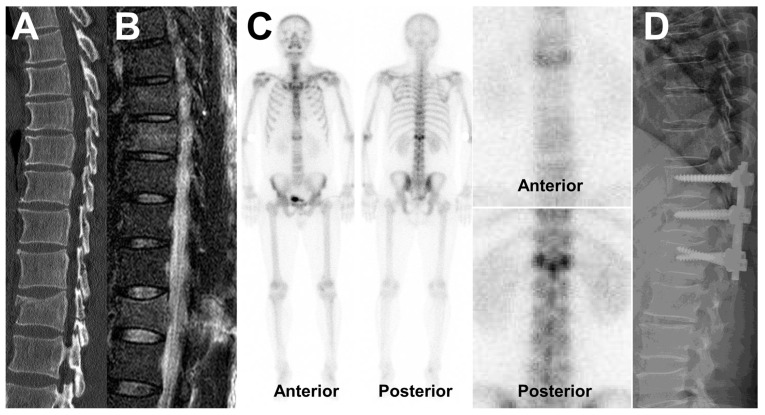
Representative images of a burst fracture of T12 in a 44-year-old patient. (A) Computed tomography and (B) magnetic resonance images show a T12 burst fracture with damage to the posterior elements of the vertebra. The TLICS was calculated as 7. (C) Bone scan images; analysis revealed that B_B_ was 3, B_P_ was 2, and their sum (B_TS_) was 5. The fracture was assessed as unstable and (D) the patient underwent posterolateral fusion at the level of T11-L1.
